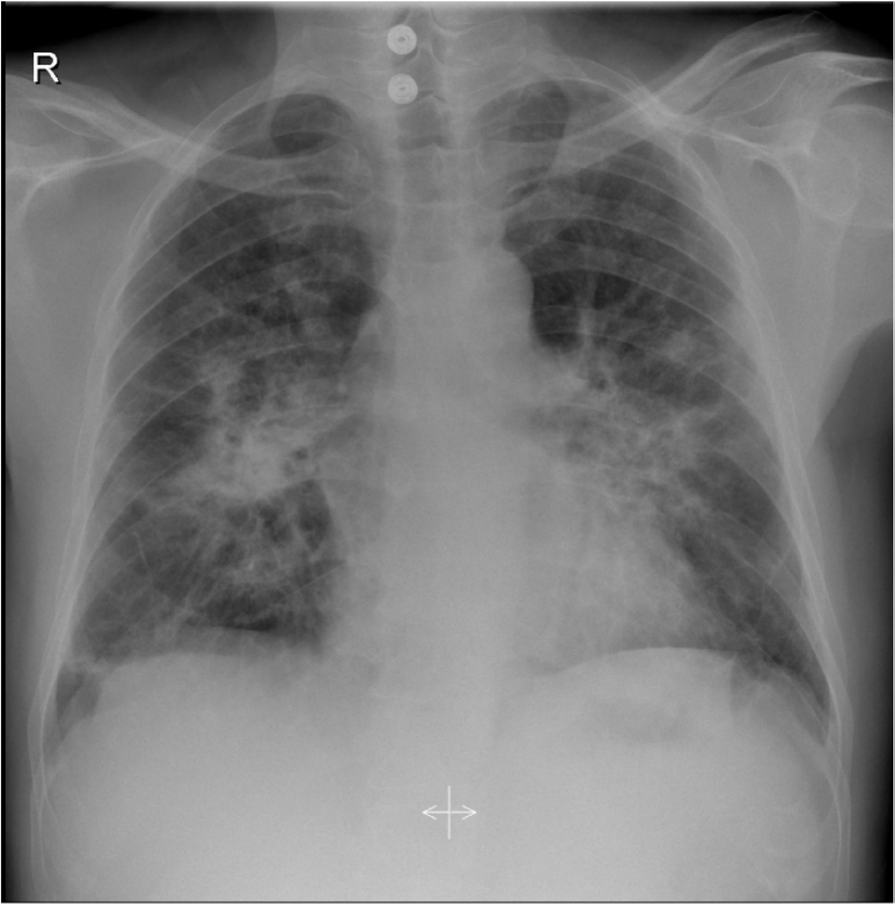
Chest X-ray showing worsening of the previously noted bilateral patchy infiltration upon recurrent admission
